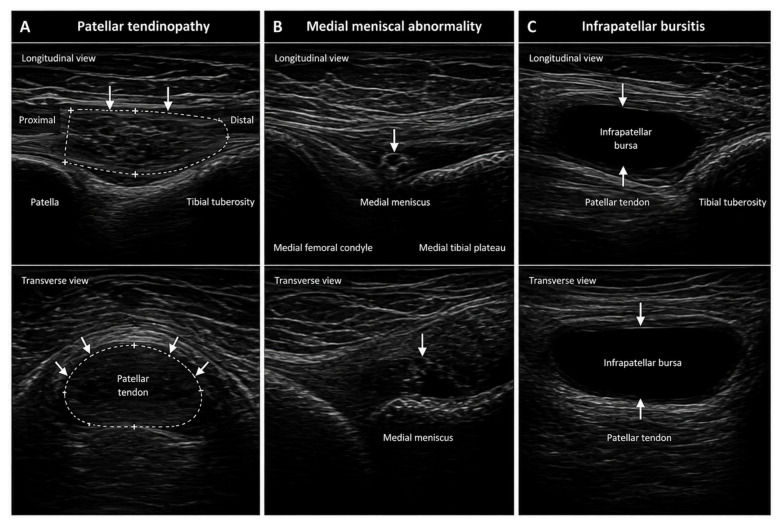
Representative ultrasonographic findings identified in the study population. (A) Patellar tendinopathy: longitudinal and transverse views demonstrating tendon thickening, focal hypoechogenicity, and loss of normal fibrillar architecture (arrows). (B) Medial meniscal abnormality: longitudinal and transverse views showing focal disruption and irregular morphology of the medial meniscus (arrows). (C) Infrapatellar bursitis: longitudinal and transverse views demonstrating abnormal anechoic fluid accumulation within the infrapatellar bursa (arrows).
